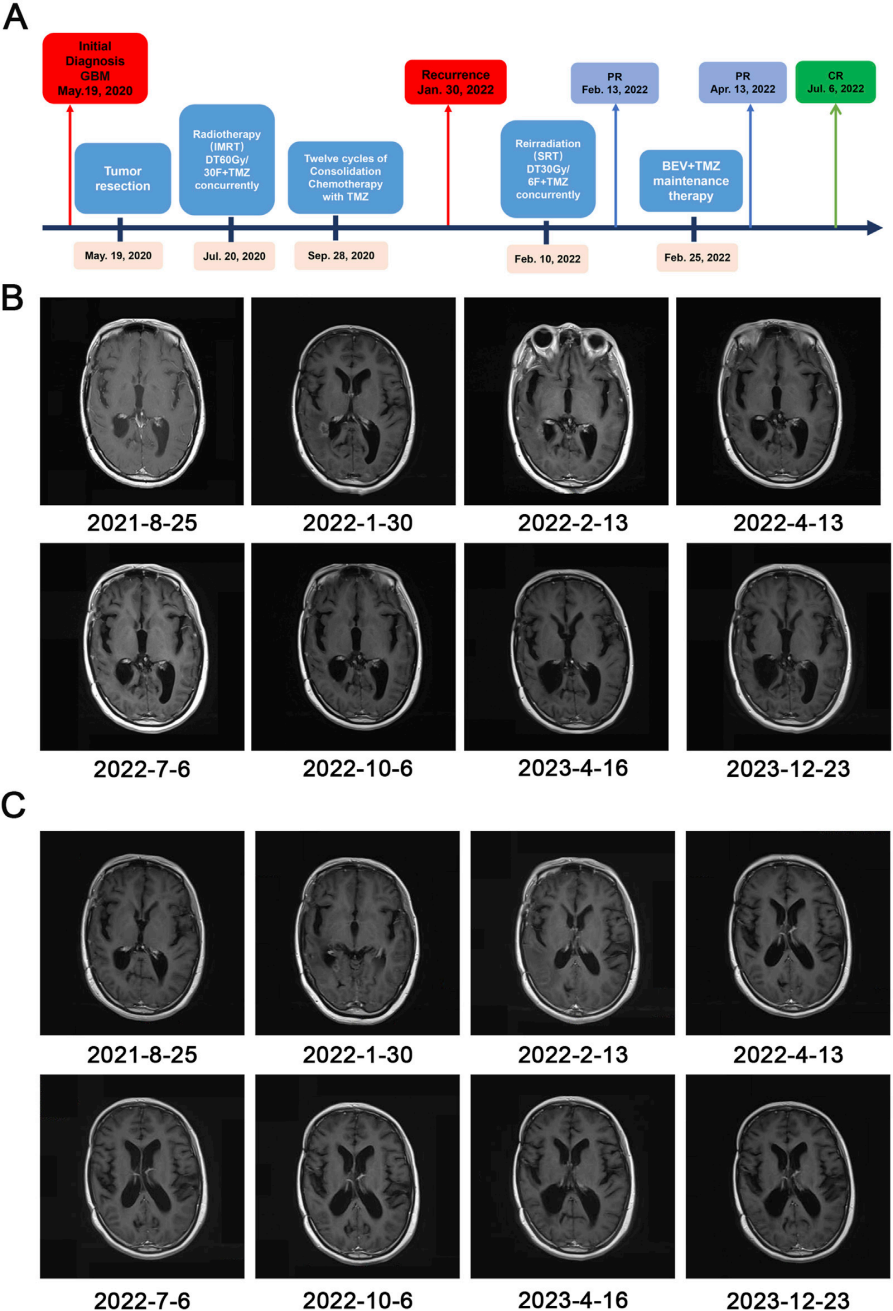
Timeline of treatment and radiographic responses. (A) Timeline of treatment. Abbreviations: Glioblastoma (GBM), partial response (PR), complete response (CR), bevacizumab (BEV), temozolomide (TMZ), stereotactic radiotherapy (SRT); (B, C) The post-contrast T1-weighted MRI showing regression of the lesion and demonstrating the effectiveness of SRT followed by bevacizumab (BEV) and temozolomide (TMZ).
